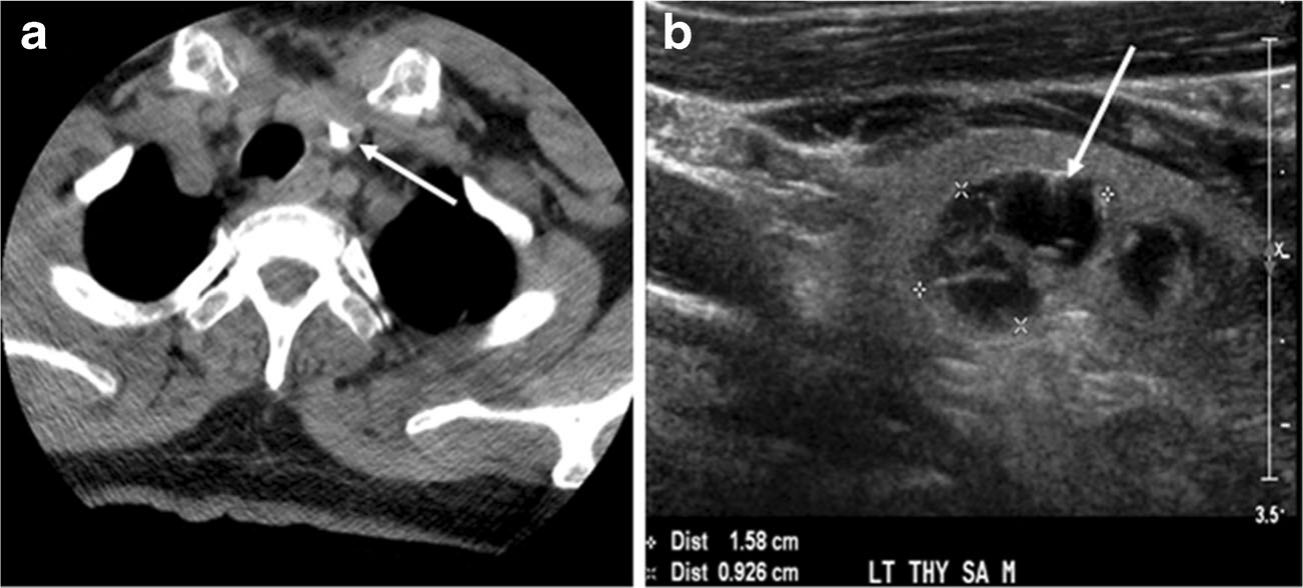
An incidentally discovered colloid nodule with calcification, shown on CT scan of a 58-year-old female patient. a Non-enhanced axial CT scan of the neck demonstrates a coarse calcification at the left thyroid inferior pole. b Sagittal grey scale ultrasound of the thyroid demonstrates a heterogeneous nodule with predominant cystic component. Calcification was not seen in the ultrasound, probably due to its lower location in the superior mediastinum
